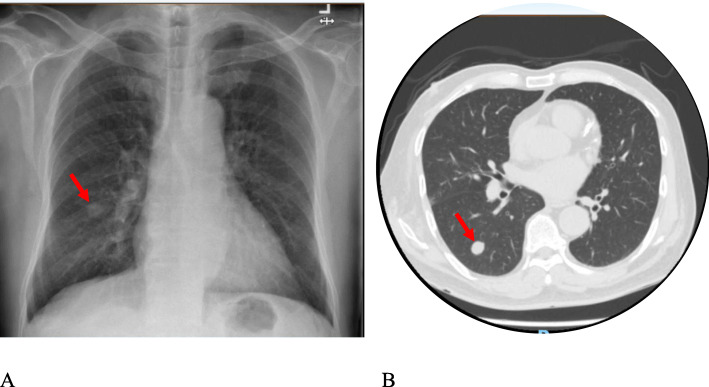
a chest x-ray and (b) CT Thorax
